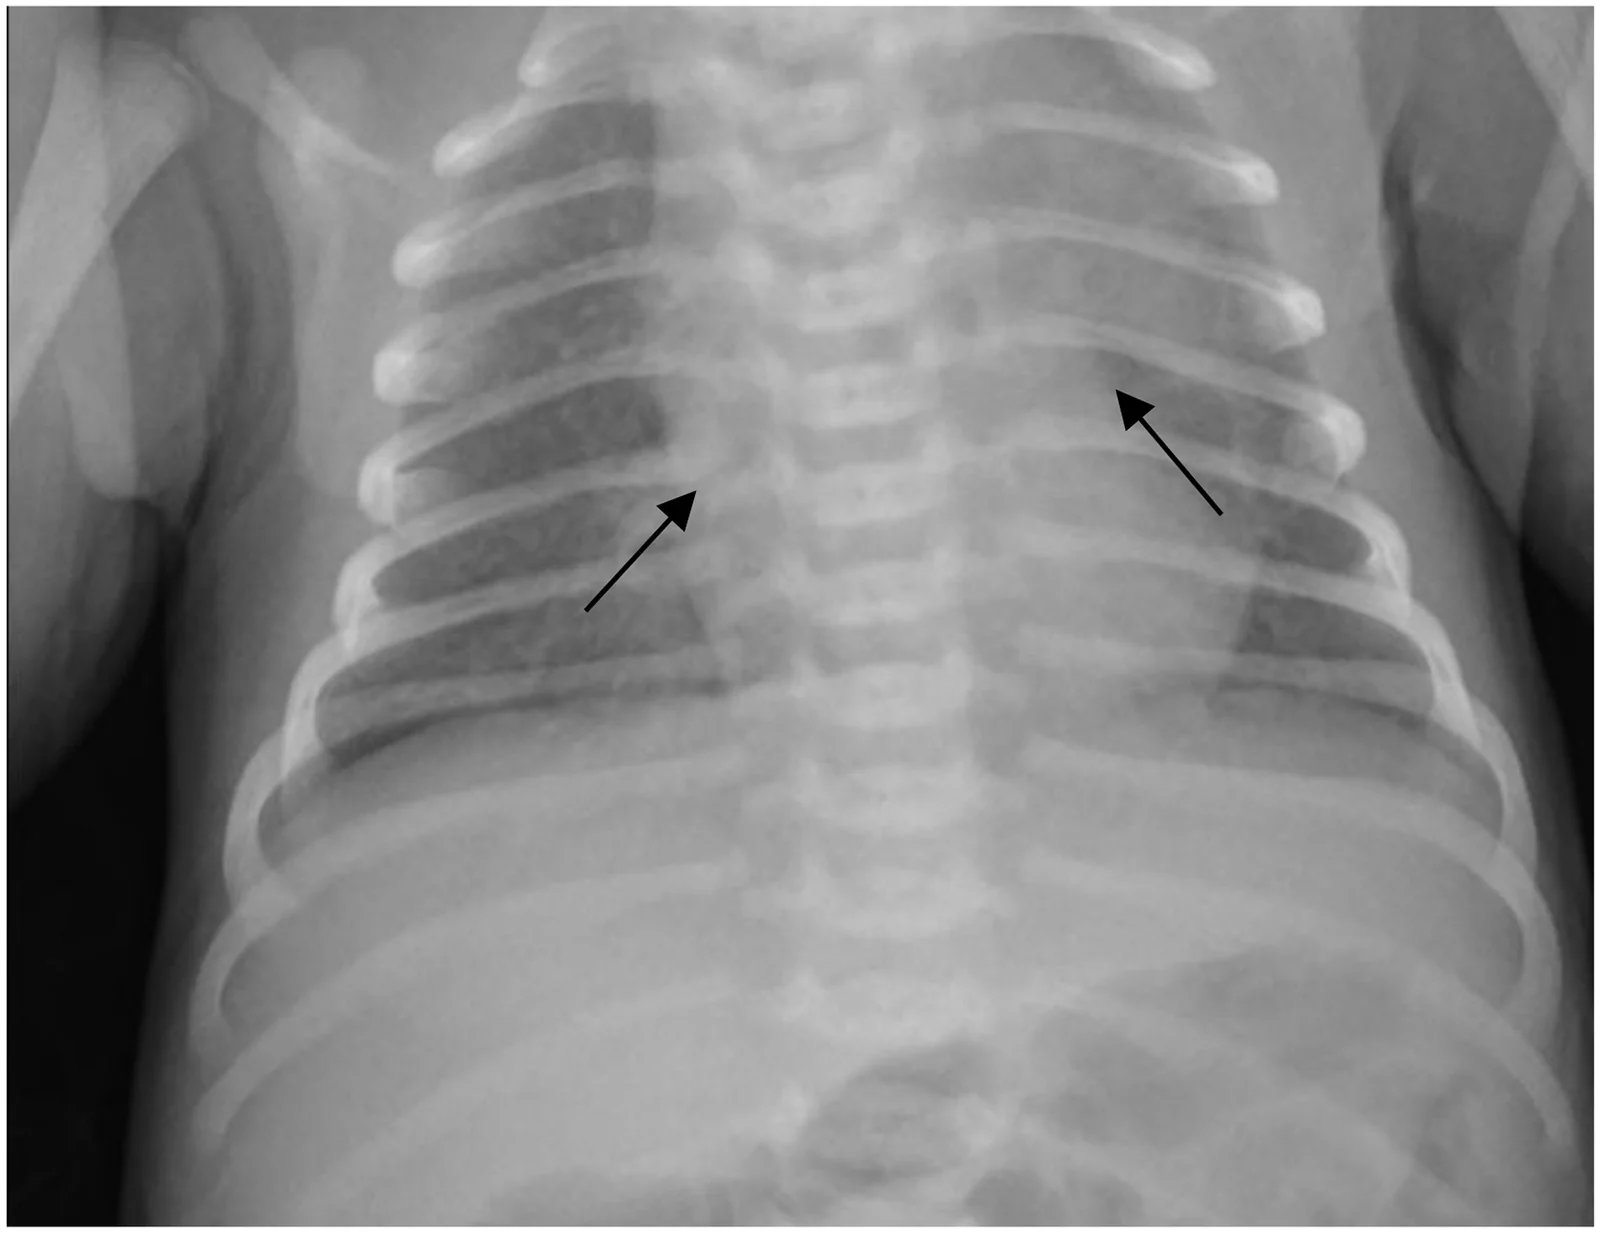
Follow-up chest radiograph obtained on day 7 of hospitalization demonstrating complete resolution of the pneumomediastinum and normalization of thymic contour (arrows).
